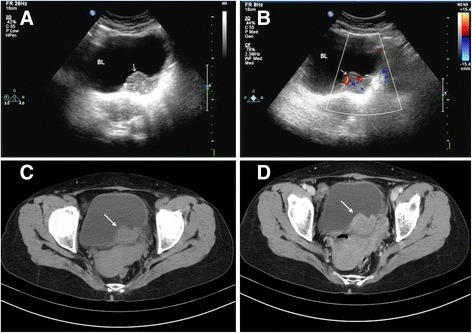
Abdominal ultrasound examination indicates a 43×28 mm slightly enhanced echo on the *left* side of the bladder wall (a). Color Doppler Flow Imaging detects a strip-like blood flow signal and records the pulsatile flow spectrum (b). Pelvic-enhanced computed tomography examination shows nodular soft tissue shadow at the left posterior wall of the bladder (c), which was obviously enriched after enhancement (d)
